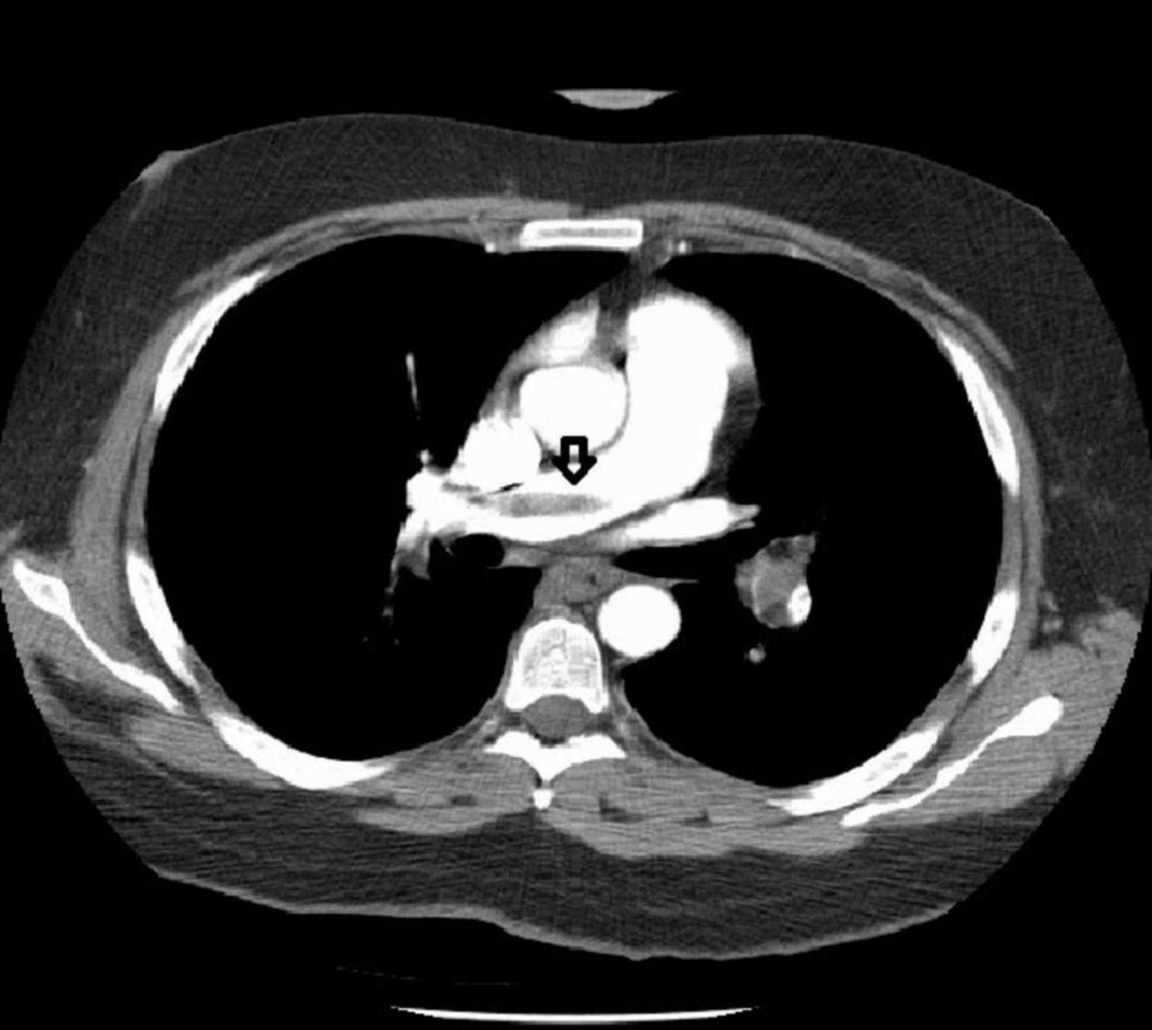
CT pulmonary angiogram showing embolus extending into the right main trunk
